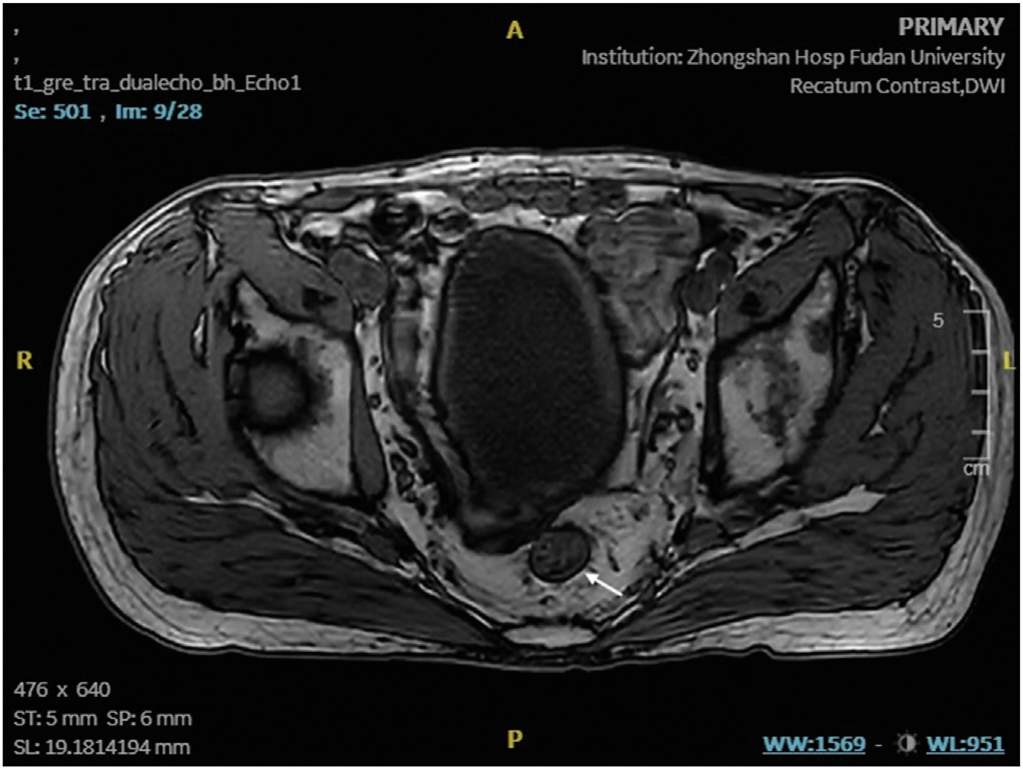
Magnetic resonance imaging demonstrating the downstage of the rectal cancer (*white arrow*).
